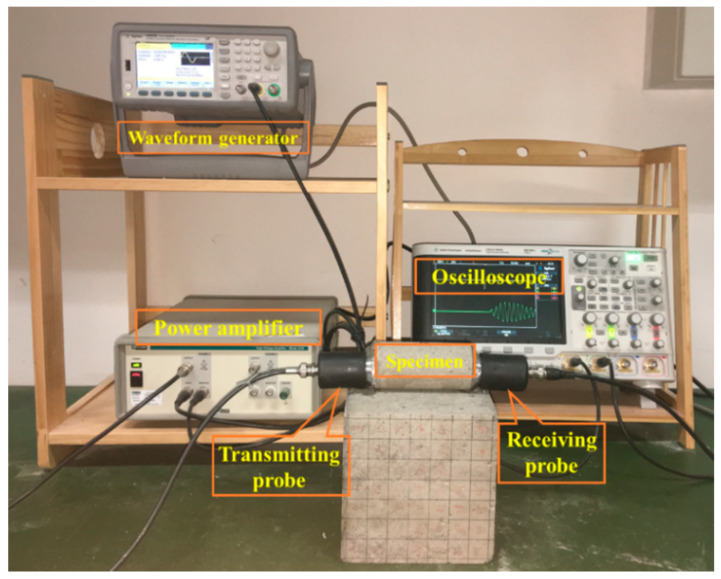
Ultrasonic testing setup.
